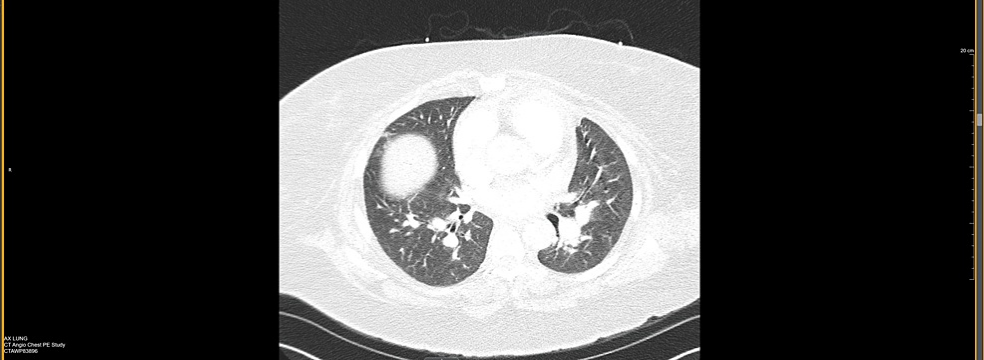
Computed tomography scan of chest angiogram in transverse section reveals right-sided hemidiaphragmatic elevation
